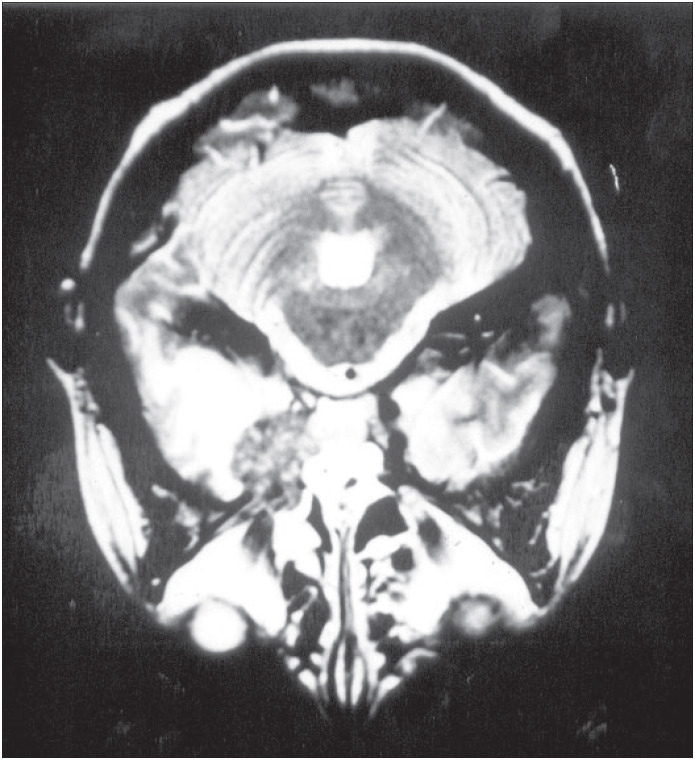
Magnetic resonance imaging of the brain in the T2 series, showing left temporal lobe edema.
